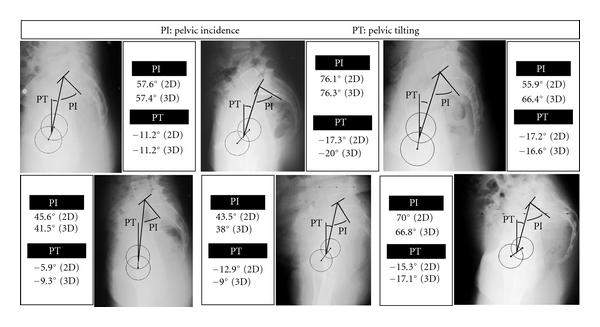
The sagittal X-ray of the standing patient does not represent a pure profile of the pelvis: the hip axis *Y*
